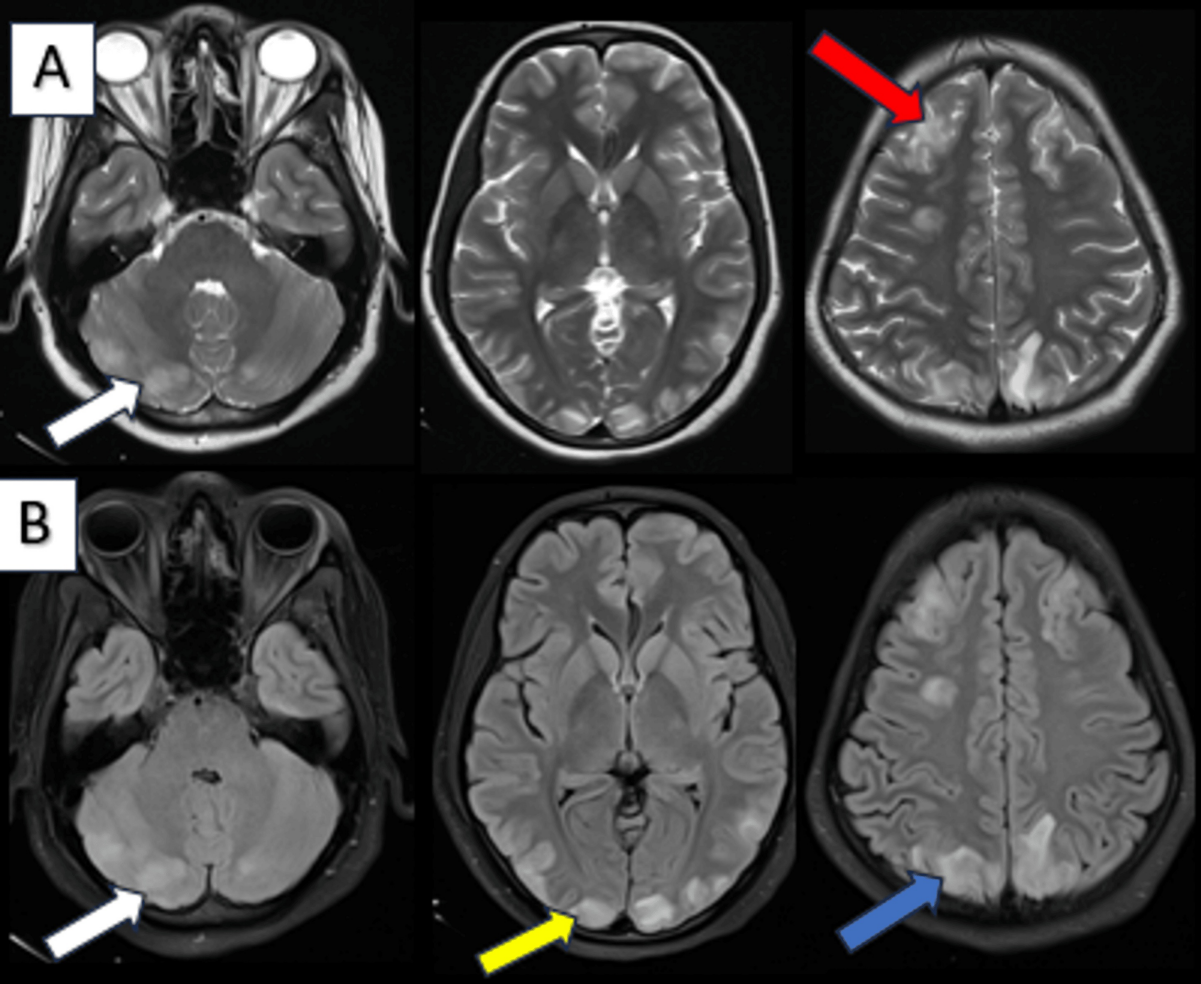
Axial T2W (A) and FLAIR (B) brain MRI. A 20-year-old female presented with seizures and altered sensorium diagnosed de novo as young onset hypertension. Axial MRI of the brain showed T2W/FLAIR hyperintensities in the subcortical white matter of the bilateral frontal (red arrow), parietal (blue arrow), and occipital (yellow arrow) lobes and the bilateral cerebellar hemispheres (white arrow). T2W: T2-weighted; FLAIR: fluid-attenuated inversion recovery.
